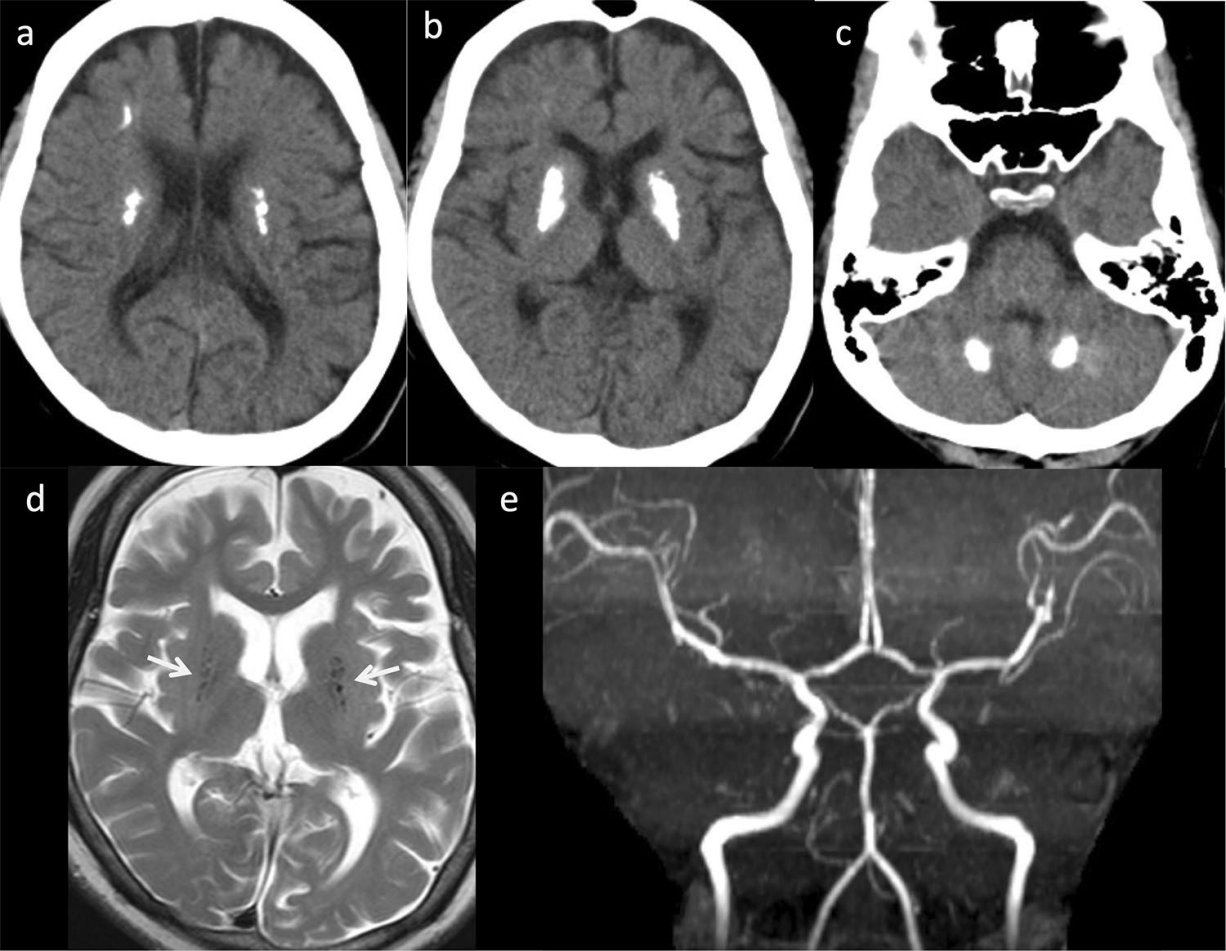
Aicardi-Goutieres syndrome with *IFIH1* mutations in a 15-year-old, female patient with quadriplegia and chilblain skin lesion of the big toe. a–c Axial non-contrast computed tomography found multiple calcifications of the bilateral, right frontal, subcortical white matter, bilateral deep white matter, basal ganglia, and cerebellar dentate nuclei. d Axial T2-weighted imaging demonstrated mild cerebral atrophy. The bilateral basal ganglia demonstrated a few, punctate hypointensities secondary to calcification (arrows). e MR angiography revealed mild stenosis of the major cerebral arteries
